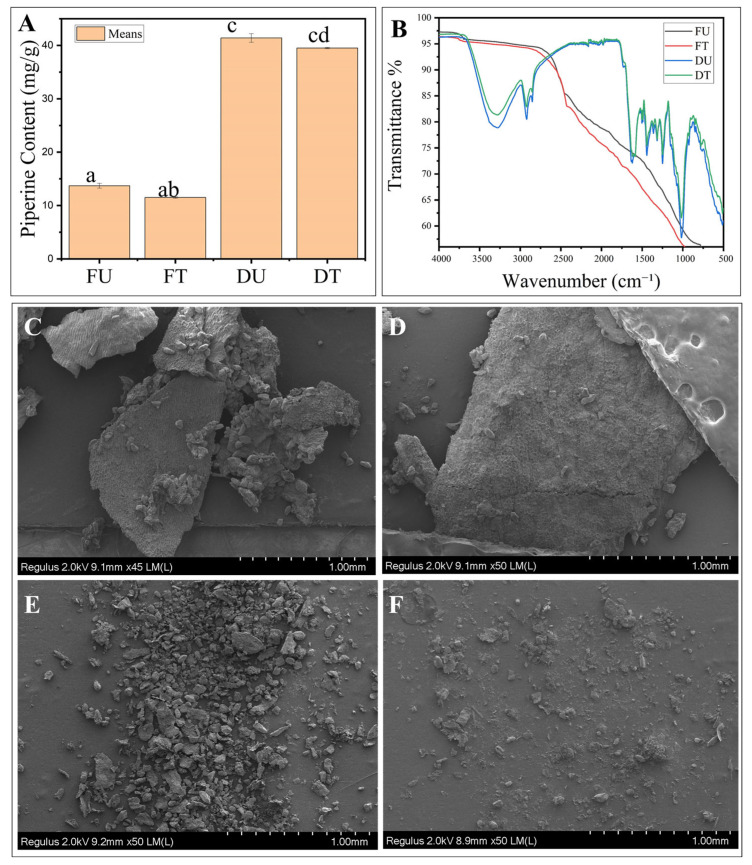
Bioactive compound preservation analysis. (A) HPLC piperine quantification showing: fresh untreated (FU: 13.70 ± 0.45 mg/g DW), fresh treated (FT: 11.50 ± 0.14 mg/g DW), dried untreated (DU: 41.80 ± 0.68 mg/g DW), and dried treated (DT: 39.51 ± 0.12 mg/g DW). Different letters (a–d) indicate significant differences (*p* < 0.05, Tukey’s HSD test). Data represent mean ± SD (n = 3). (B) FTIR spectra (4000–500 cm^−1^) showing preserved molecular structure after ultrasound treatment: FU (fresh untreated), FT (fresh treated), DU (dried untreated), DT (dried treated). Microstructural SEM images showing (C) fresh untreated, (D) fresh treated, (E) dried untreated, (F) dried treated. Scale bars represent 100 μm.
